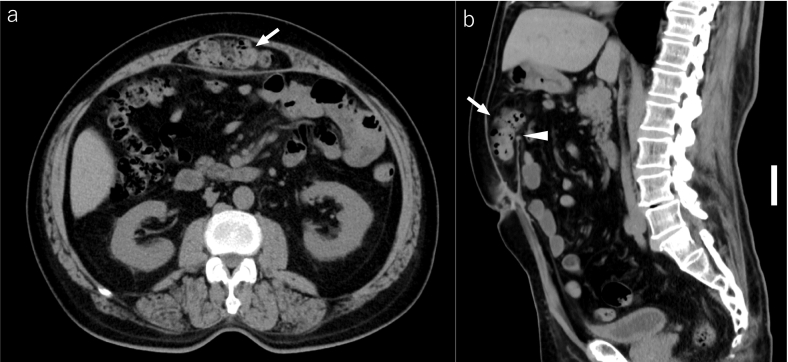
Abdominal computed tomography one year after enhanced-view totally extraperitoneal repair. a) Axial image shows the large bowel (arrow) in the retrorectus space. b) Sagittal image shows the large bowel (arrow) entering the defect of the posterior rectus sheath. An arrowhead indicates the upper edge of the mesh. There is no evidence of bowel obstruction and dehiscence of the anterior rectus sheaths. Scale bar, 5 cm.
